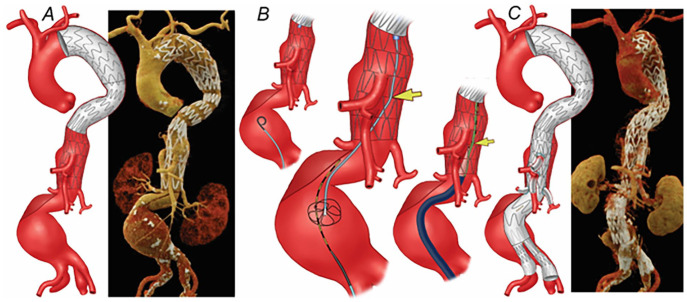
(A) Preoperative 3-dimensional computed tomography angiography (CTA) reconstruction shows a 6.8 cm extent II thoracoabdominal aortic aneurysm. A 4-vessel patient-specific fenestrated-branched stent-graft was designed for the patient. (B) During catheterization of the thoracic stent, the wire was noticed to be placed beneath one of the stent struts of the bare-metal stent, which prevented the introduction of the main graft. The same technique as described in Figure 1 was used for the catheterization of thoracic bare-metal stent. (C) Postoperative 3-dimensional CTA reconstruction showing the endovascular repair of the thoracoabdominal aortic aneurysm with no kink, stent compression, and endoleak.
